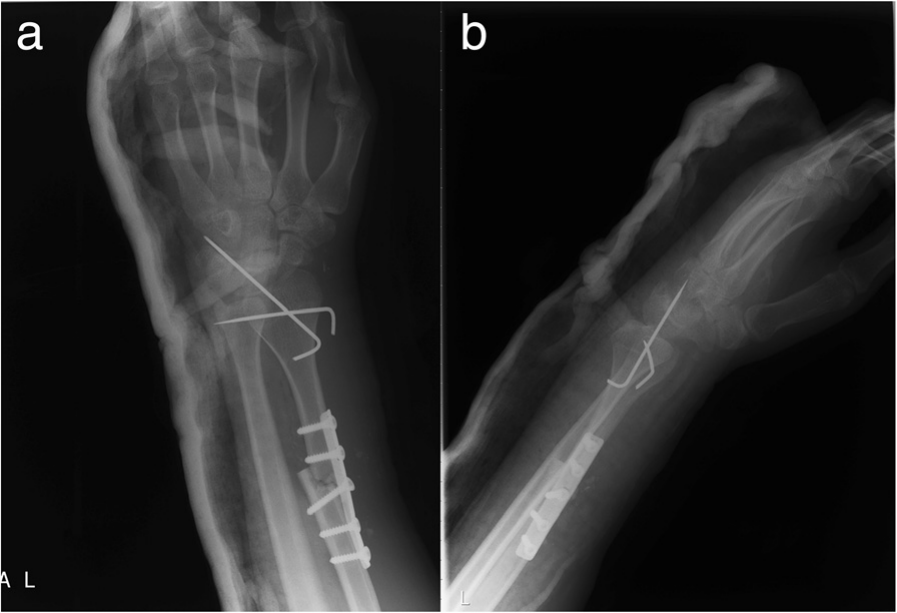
Anteroposterior and lateral X-ray images taken 1 week after en-bloc resection of RGCT and wrist reconstruction with a vascularized proximal fibular autograft (a, b)
